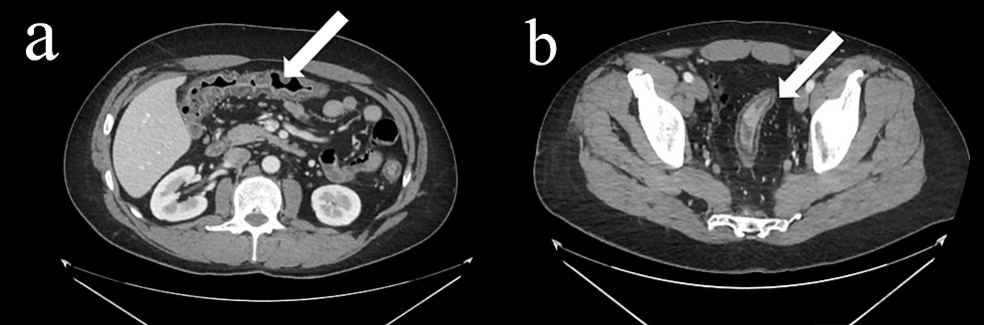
Axial Computerized Tomography (CT) images of Abdomen/Pelvis With Contrast (a) Axial computerized tomography scan images of abdomen/pelvis with contrast indicating shortening and loss of haustra, as well as submucosal fat infiltration consistent with colonic inflammation and ulcerative colitis. (b) Axial computerized tomography scan images of abdomen/pelvis with contrast indicating shortening and loss of haustra further down the abdomen indicating complete consumption of colon with inflammation.
